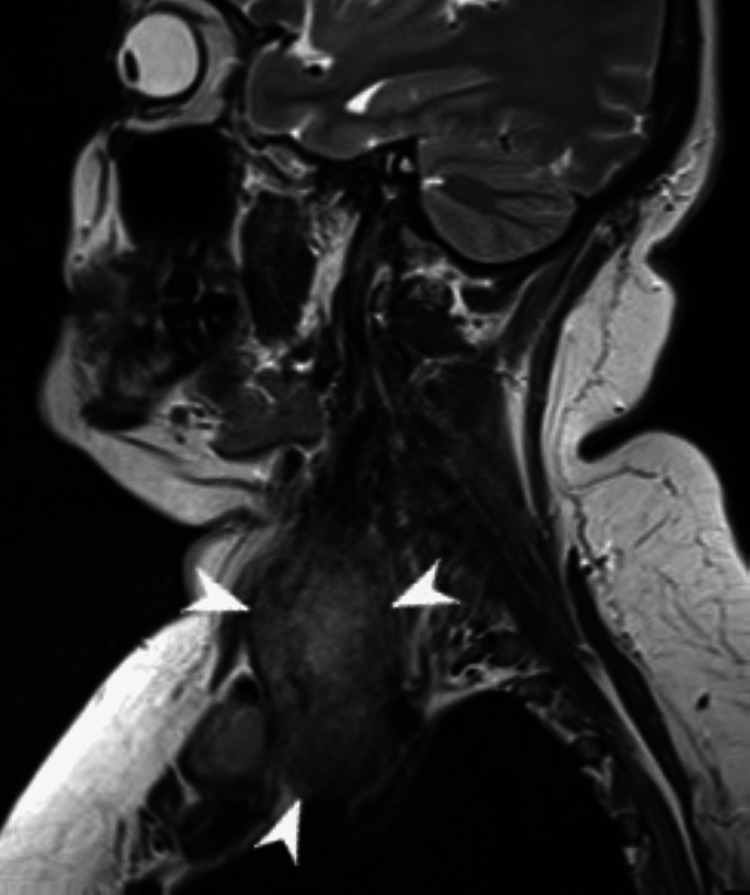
MRI neck without contrast images demonstrating a large heterogeneous mass arising from the right thyroid lobe.
